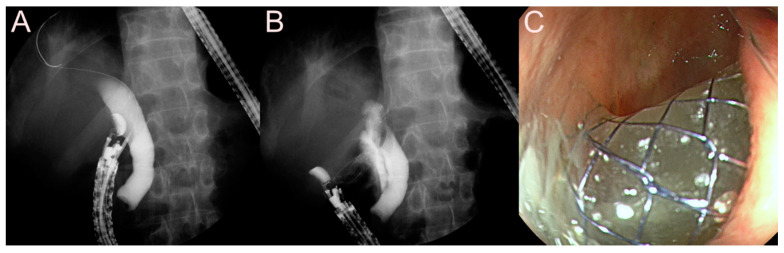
Ultrasound-guided choledochoduodenostomy using the bi-flanged, fully covered self-expandable metal stent (FCSEMS) (BONA-AL Stent; Standard Sci Tech Inc., Seoul, Korea) for distal malignant biliary obstruction. (A) Fluoroscopic image showing a guidewire delivered to the intrahepatic duct through a 19-gauge needle punctured into the common bile duct (BD). (B) Fluoroscopic image showing the bi-flanged FCSEMS placed between the duodenum and the common BD. (C) Endoscopic image showing the drainage of pus-like materials through the bi-flanged FCSEMS.
